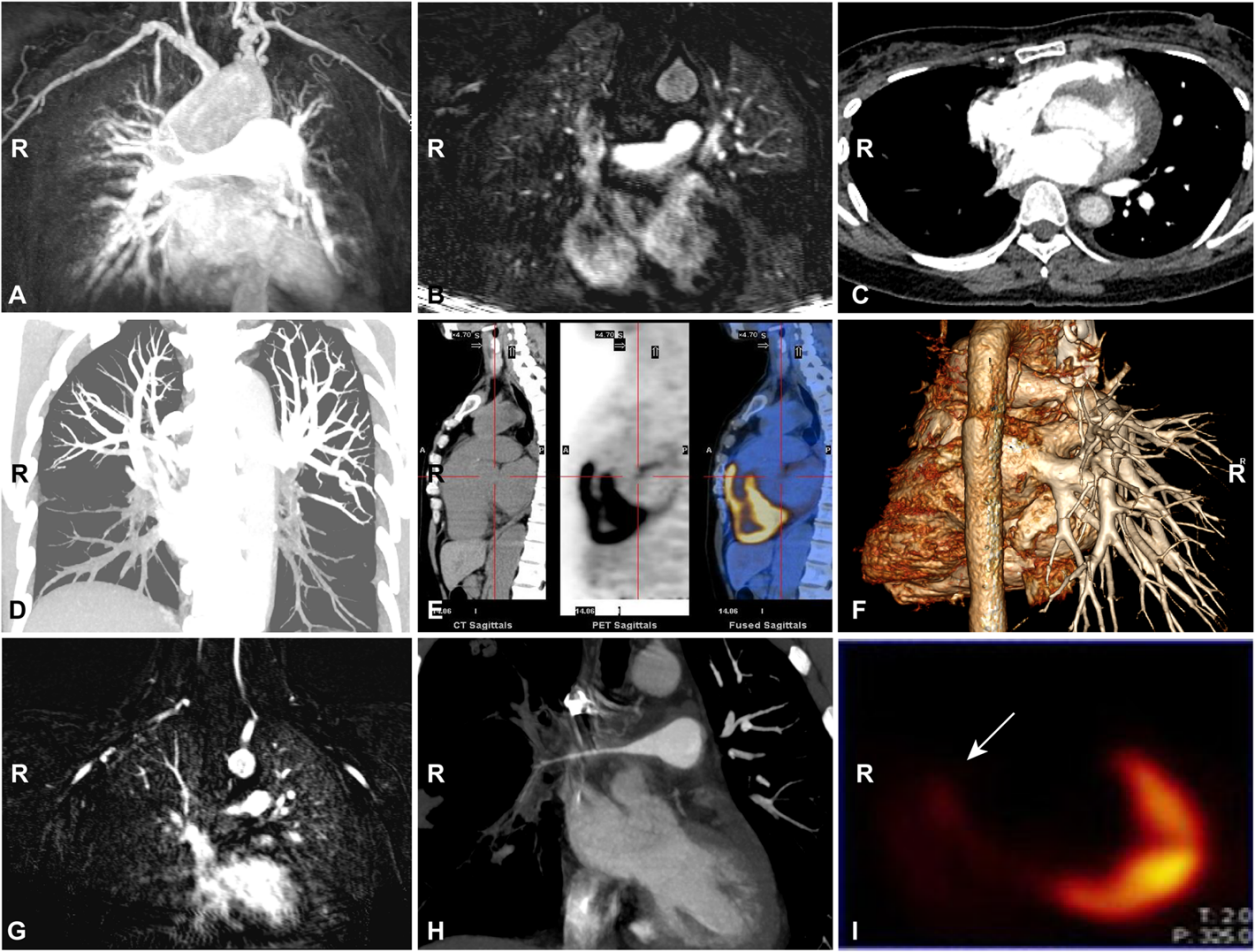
Imaging of PA lesions in TA patients. a Dilation of the PT upon CTA. b Thickness of the PT upon MRA. c Stenosis of the right main PA upon CTA. d Thrombosis of the lower PAs on both sides upon CTA. e Inflammation of the pulmonary trunk root upon PET-CT (SUV 4.1). f The absence of left PAs and stenosis of the right main PA (reconstructed image of CTA). Pulmonary MRA (g), CTA (h), and VQ scan (i) of a patient with TA. MRA shows a fine right main PA and low perfusion in the right lung (g). CTA demonstrates a fine right main PA and fewer PA branches in the right lung (h). Lung VQ scan showed filling defect of the complete right lung (i)
